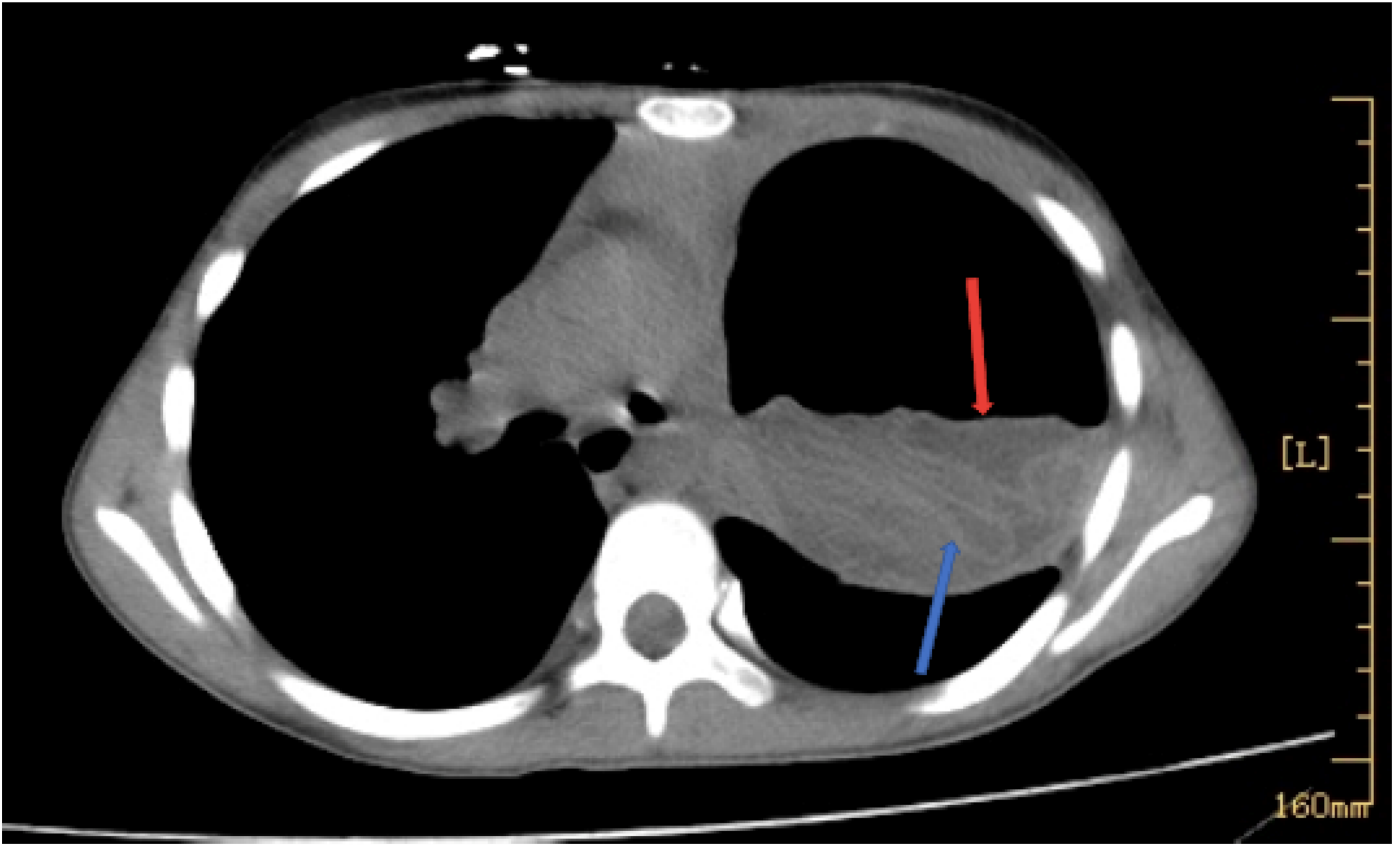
Image of the chest CT. The images revealed cystic liquid shadow (the red arrow), and whirl sign or a ribbon sign (the blue arrow).
